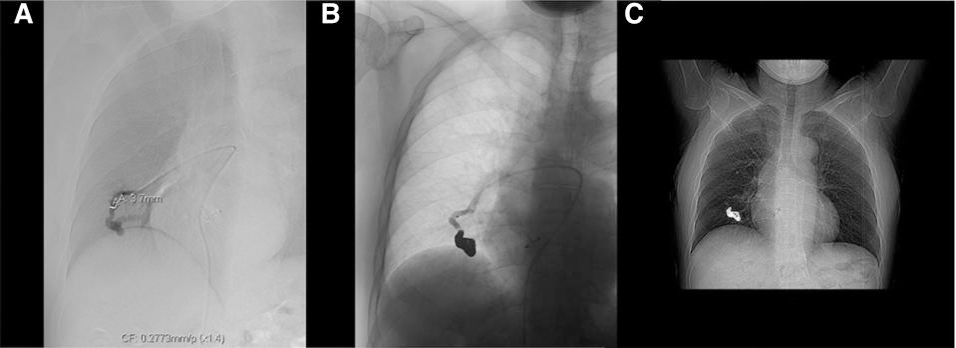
Pulmonary arteriovenous malformation closure procedure. (*A*) Selective angiography of pulmonary arteriovenous malformation before closure. (*B*) Selective angiography of pulmonary arteriovenous malformation after closure, showing no contrast passage. (*C*) Chest X-ray after pulmonary arteriovenous malformation closure.
